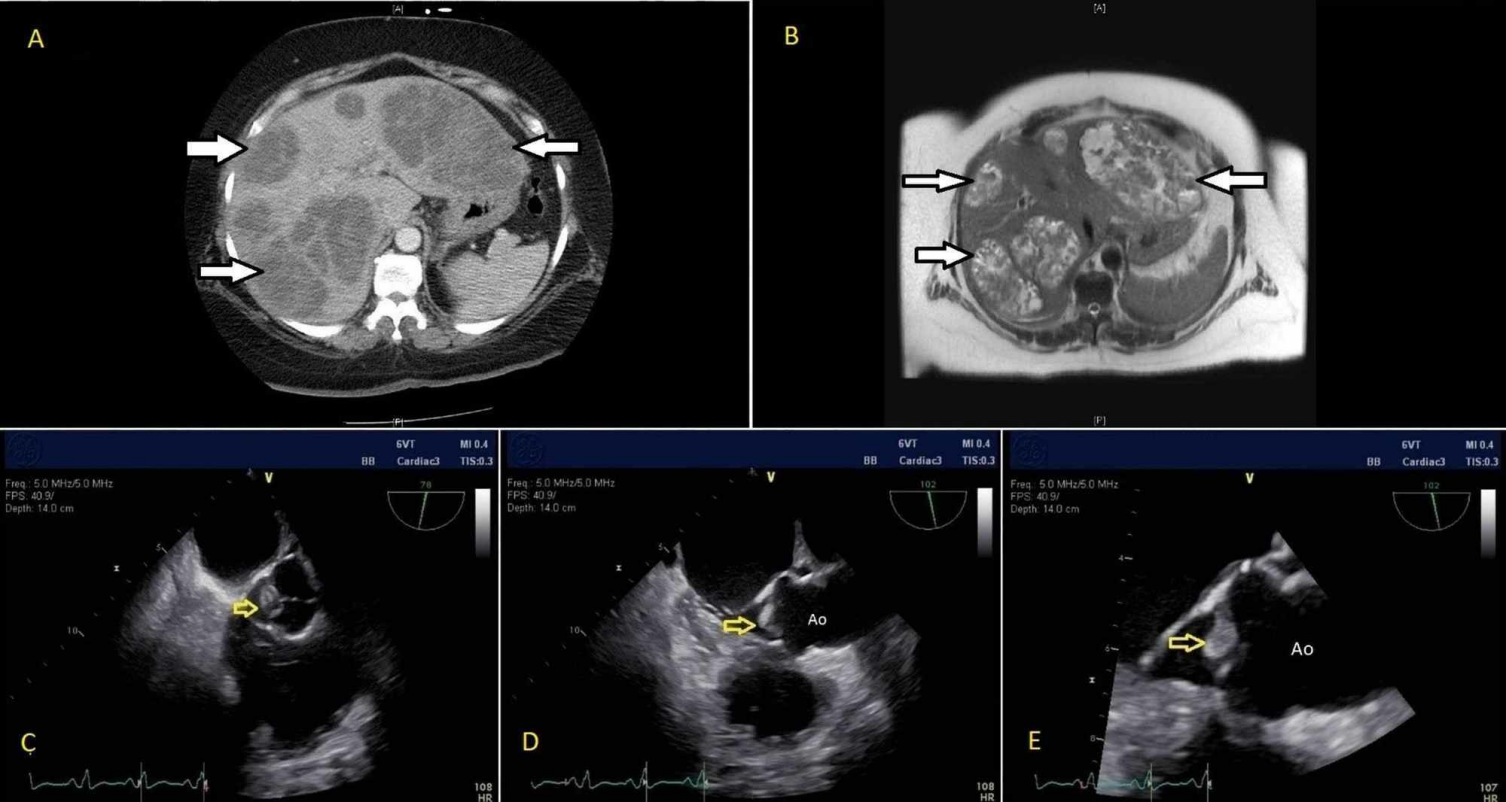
(A) CT abdomen and (B) MRCP finding of multiple hepatic abscesses (solid white arrows); (C-E) Transesophageal echocardiographic findings of aortic valvular vegetation (yellow arrows) Ao, Aorta; CT, Computed Tomography; MRCP, Magnetic Resonance Cholangiopancreatography
